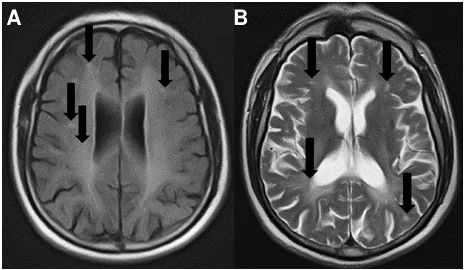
A and B show the brain magnetic resonance imaging of patient 1. Image A demonstrates periventricular predominant leukodystrophy on a FLAIR sequence, the optimal sequence for assessing white matter abnormalities. Image B reveals hyperintense findings consistent with leukodystrophy, primarily in the parieto-occipital region.
